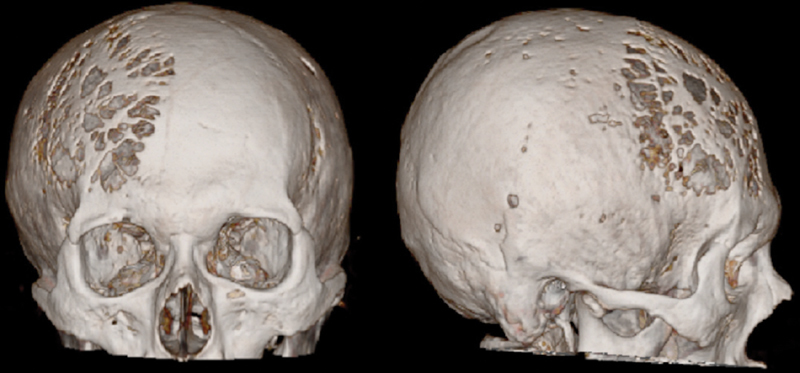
Three-dimensional reconstruction based on the computed tomography scan through a bone window on admission, showing multiple punched-out lesions of the skull.
